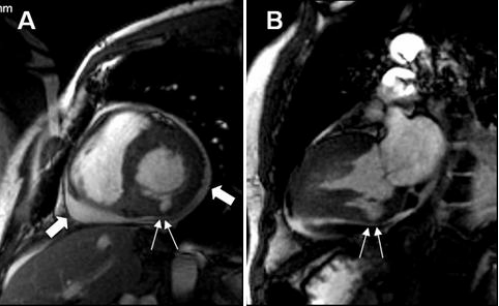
Cardiac MRI in short (A) and long (B) axis views showing pericardial effusion (large arrows) and thinning and dissection of the left ventricular inferior wall (small arrows).
